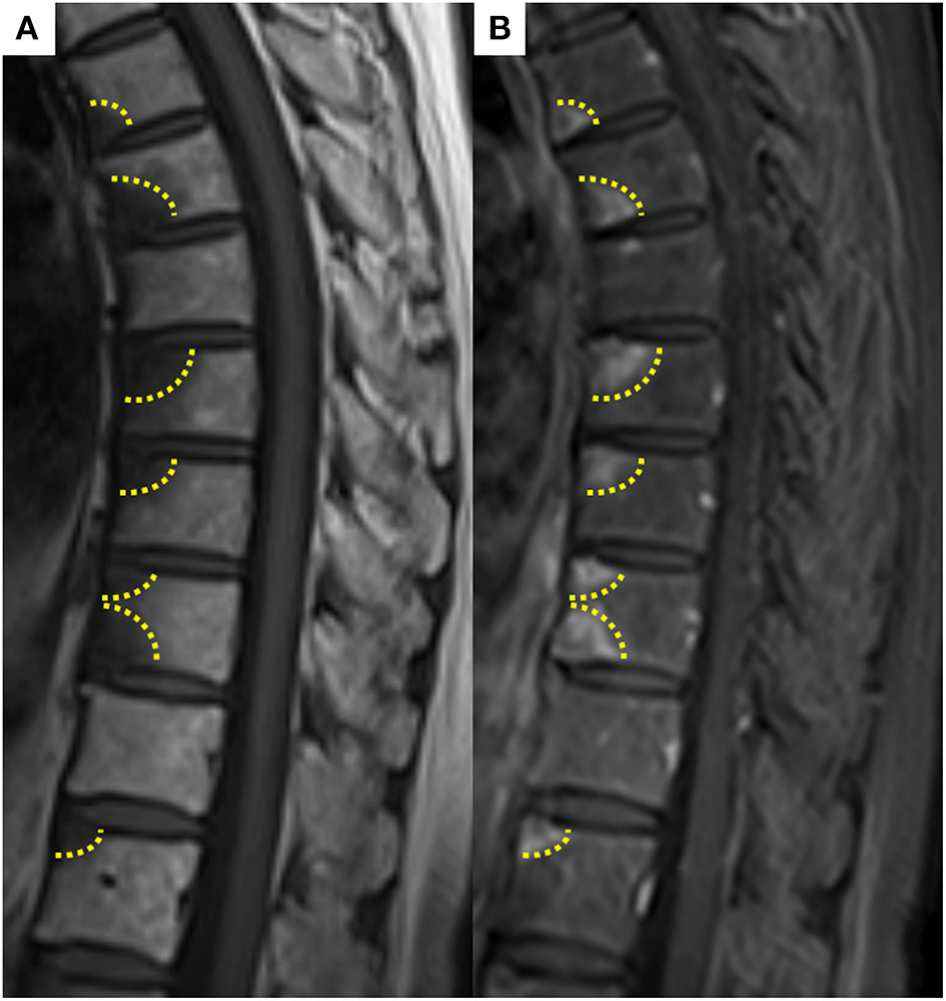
(A) Spine magnetic resonance imaging showed hypointense area on T1-weighted image, (B) abnormally enhancing effect on contrast T1-weighted image in contiguous anterior thoracic vertebral corners. These multiple vertebral lesions had a characteristic semi-circular pattern (dashed line) in contiguous vertebral body segments.
